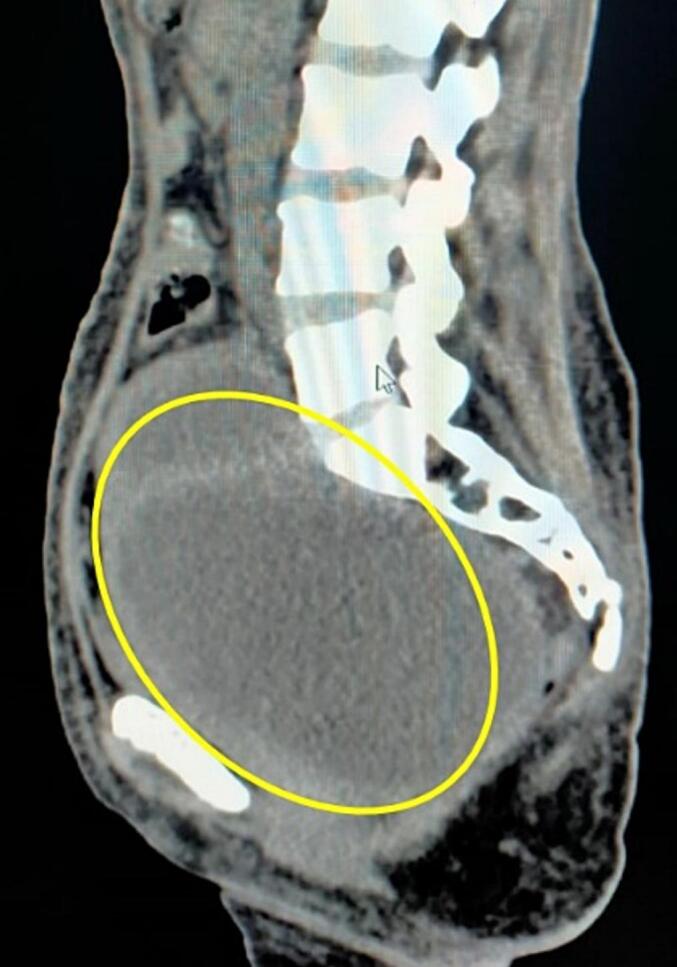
Ultrasonography (USG). Ultrasonography revealed a midline thick-walled adnexal cyst measuring 11 × 10 cm with internal septation.
